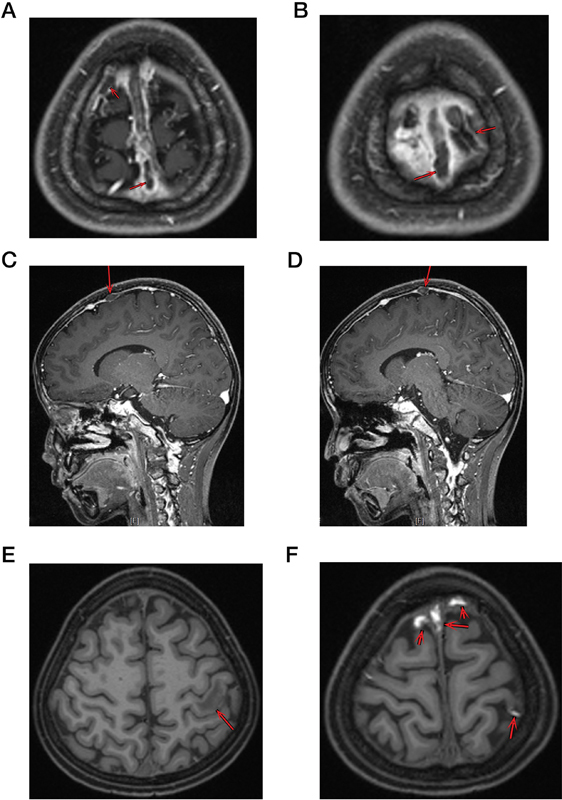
Radiographic data of first thromboses. (
